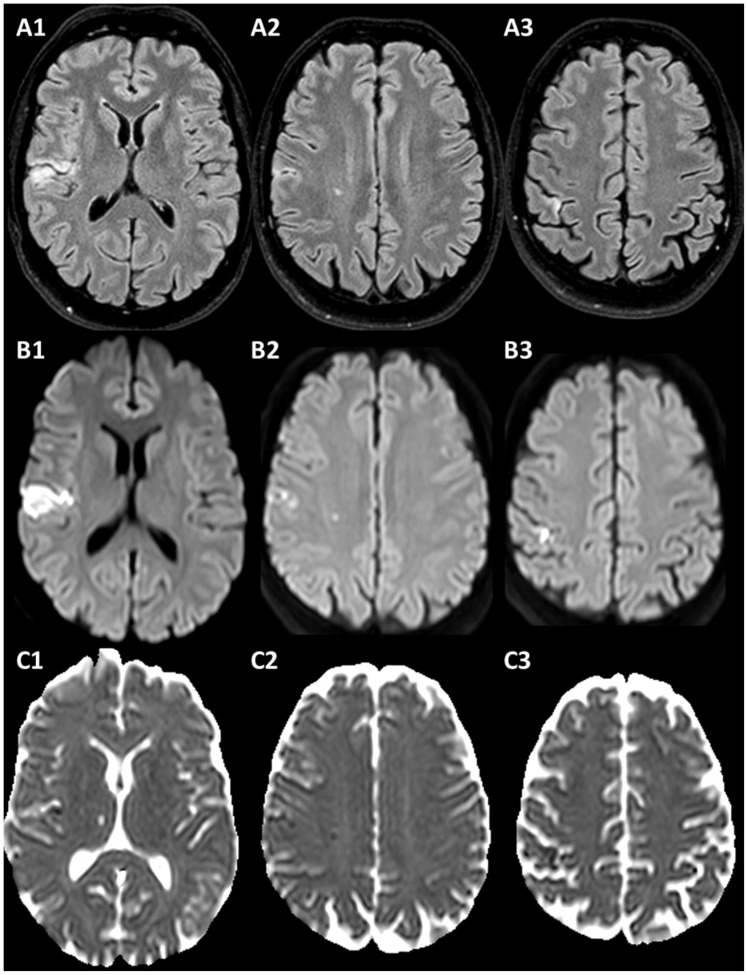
Brain MRI of Case 1. (A1–A3) are axial FLAIR slices showing multiple cortical and subcortical hyperintense areas in the right hemisphere, with corresponding DWI hyperintensity (B1–B3) and ADC decrease (C1–C3), as recent ischemic lesions.
